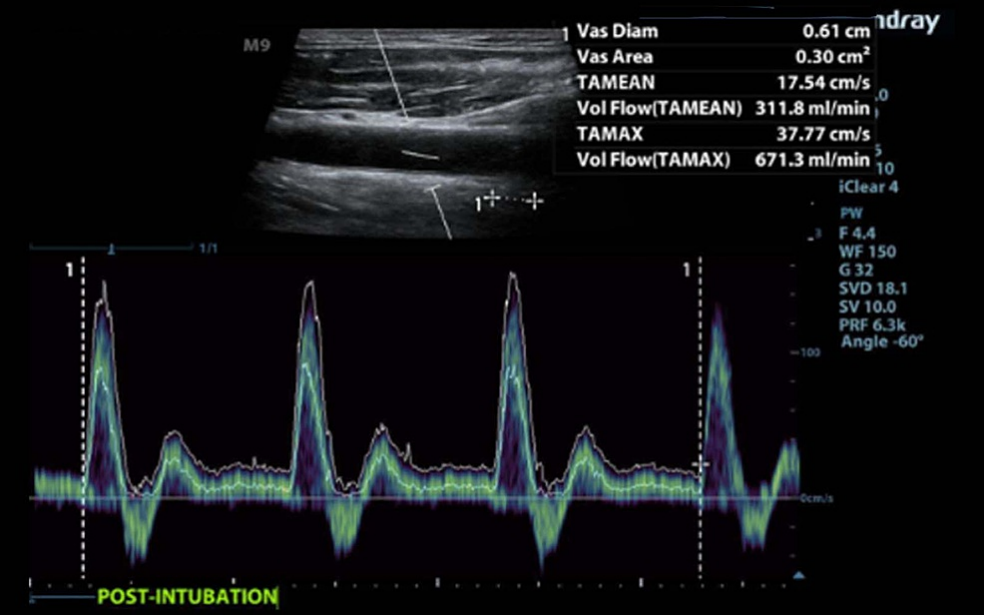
Flow Volume Pulse wave Doppler with measurement of flow volume, Time Average Mean Velocity (TAMEAN). The vascular diameter was measured in transverse at the same location as the doppler image. The diameter result was transferred to the longitudinal image so the ultrasound machine could calculate the area and the flow volume.
